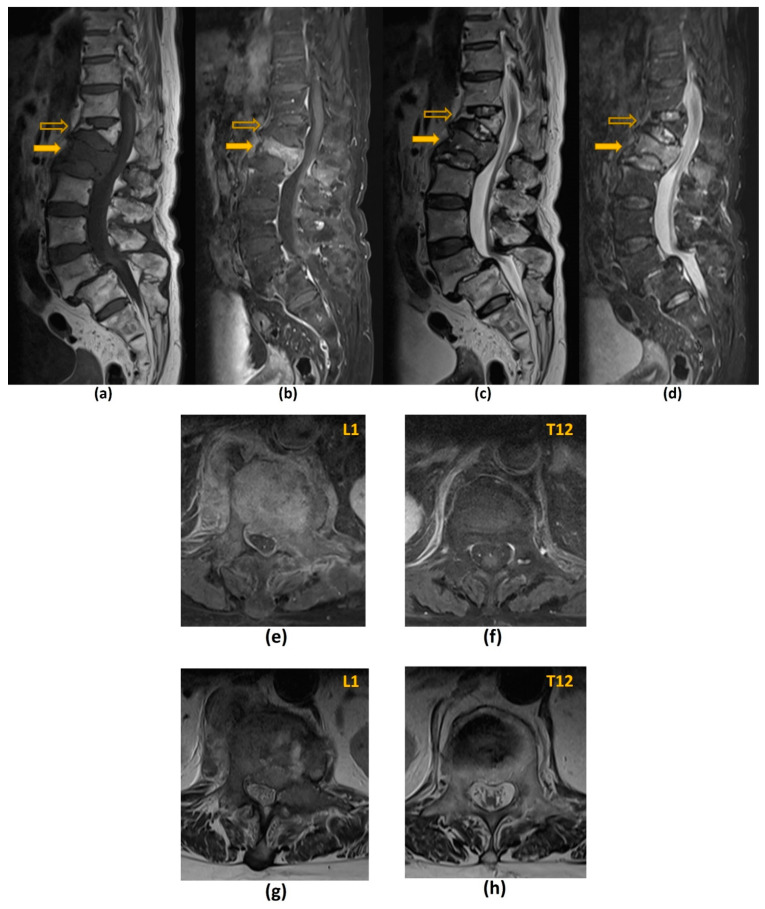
Sagittal pre-contrast T1-weighted (a), post-contrast fat-suppressed T1-weighted (b), T2-weighted (c) and STIR (d) sequences of a 70-year-old male patient with lung cancer that had metastasized to the spine. Axial post-contrast fat-suppressed T1-weighted sequences at the level of L1 (e) and T12 (f), and axial T2-weighted sequences at the level of L1 (g) and T12 (h) of the same patient. There is a pathological L1 compression fracture (solid arrow) with diffuse fatty marrow replacement extending to the posterior elements by the tumour. A convex posterior border is demonstrated as well as an enhancing epidural component. This causes low-grade (Bilsky 1c) MSCC. At the level of T12 (open arrow), no significant marrow replacement is seen and the posterior elements return normal signal intensities. There is subtle retropulsion of fracture fragments and no enhancing epidural component. These findings suggest a T12 osteoporotic compression fracture.
